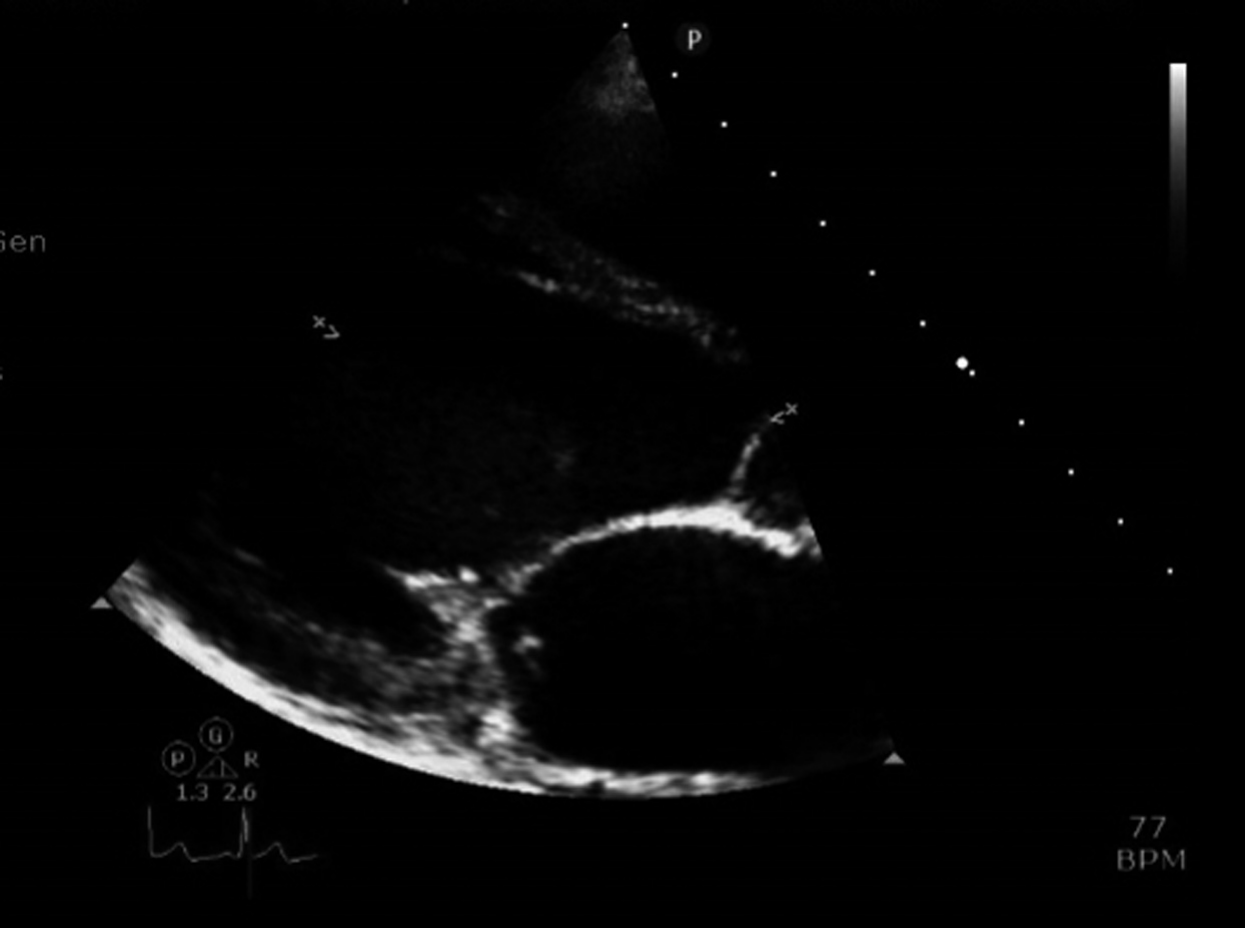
Parasternal view showing thickened mitral valve and vegetation.
